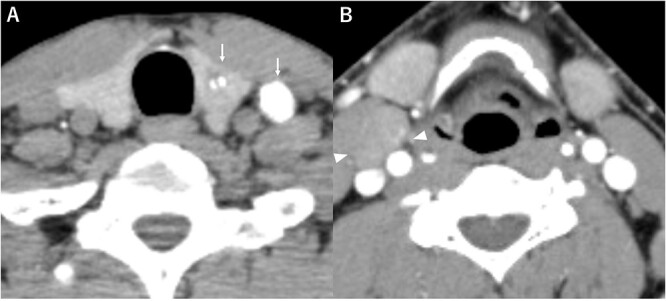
Computed tomography (A) calcified nodules in the left lobe of the thyroid and the left cervical lymph nodes (arrow), (B) the right cervical lymph node mass with no calcification or no contrast effect (arrowhead).
